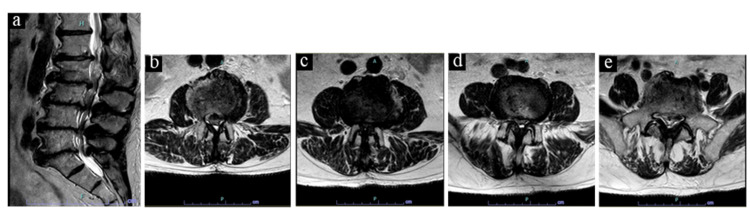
(a) Sagittal, (b) axial L2/3, (c) axial L3/4, (d) axial L4/5, and (e) axial L5/S1 non-contrast MRI images of lumbar spine preoperatively.
